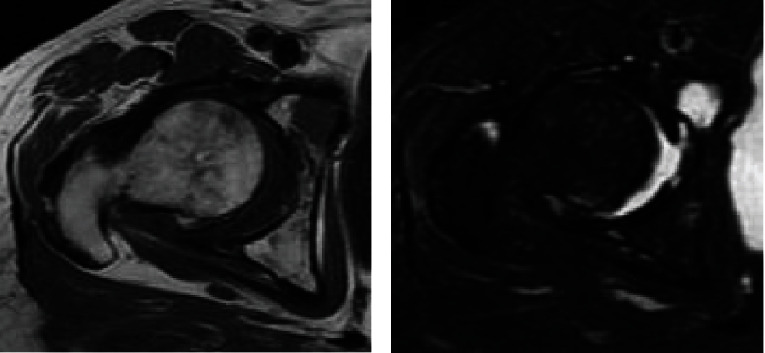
Nonenhanced (a) T1-weighted image and (b) T2-weighted image magnetic resonance image reveals multiple intraosseous lesions in the periacetabular region. There are no periacetabular pathologic fractures.
